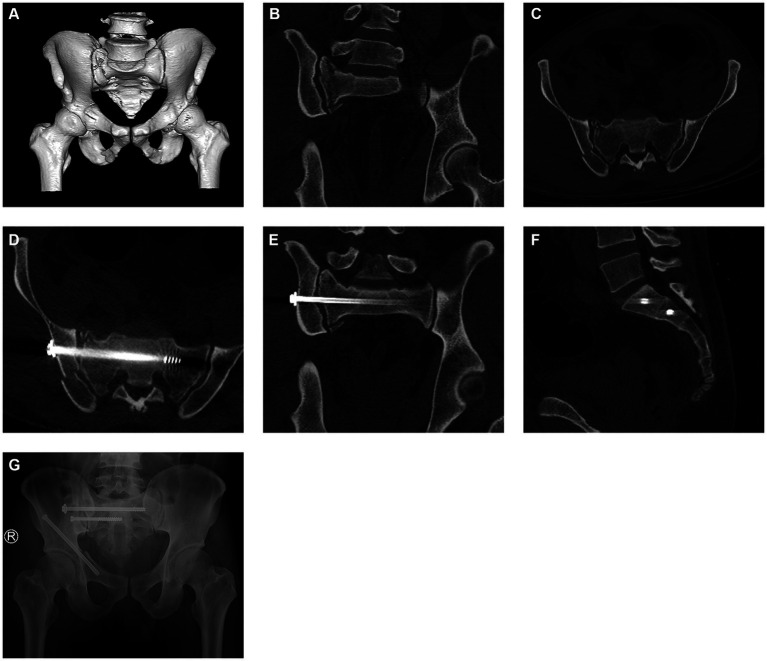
Postoperative images of the ilio-sacral screw assisted by robot. Fracture of sacrum (A–C), Position of ilio-sacral screw (D–F), Radiographs 6 months after surgery (G).
